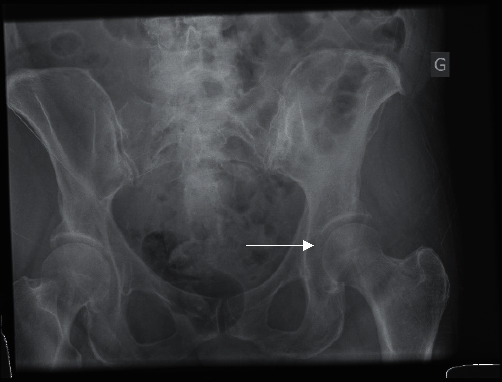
Anteroposterior radiograph after the second closed reduction. *Note*. The uneven femoroacetabular joint space on the left side (arrow).
